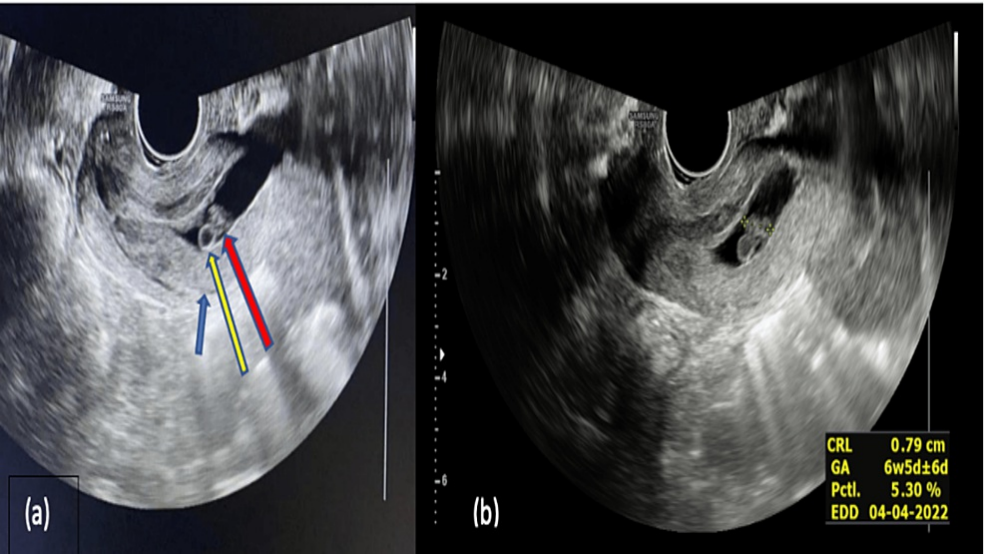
Endovaginal ultrasonography image showing the (a) cervix (blue arrow) with yolk sac (yellow arrow) and fetal pole (red arrow) within the endocervical canal, with (b) CRL of 7.9 mm corresponding to six weeks and five days of gestation. CRL: crown-rump length
